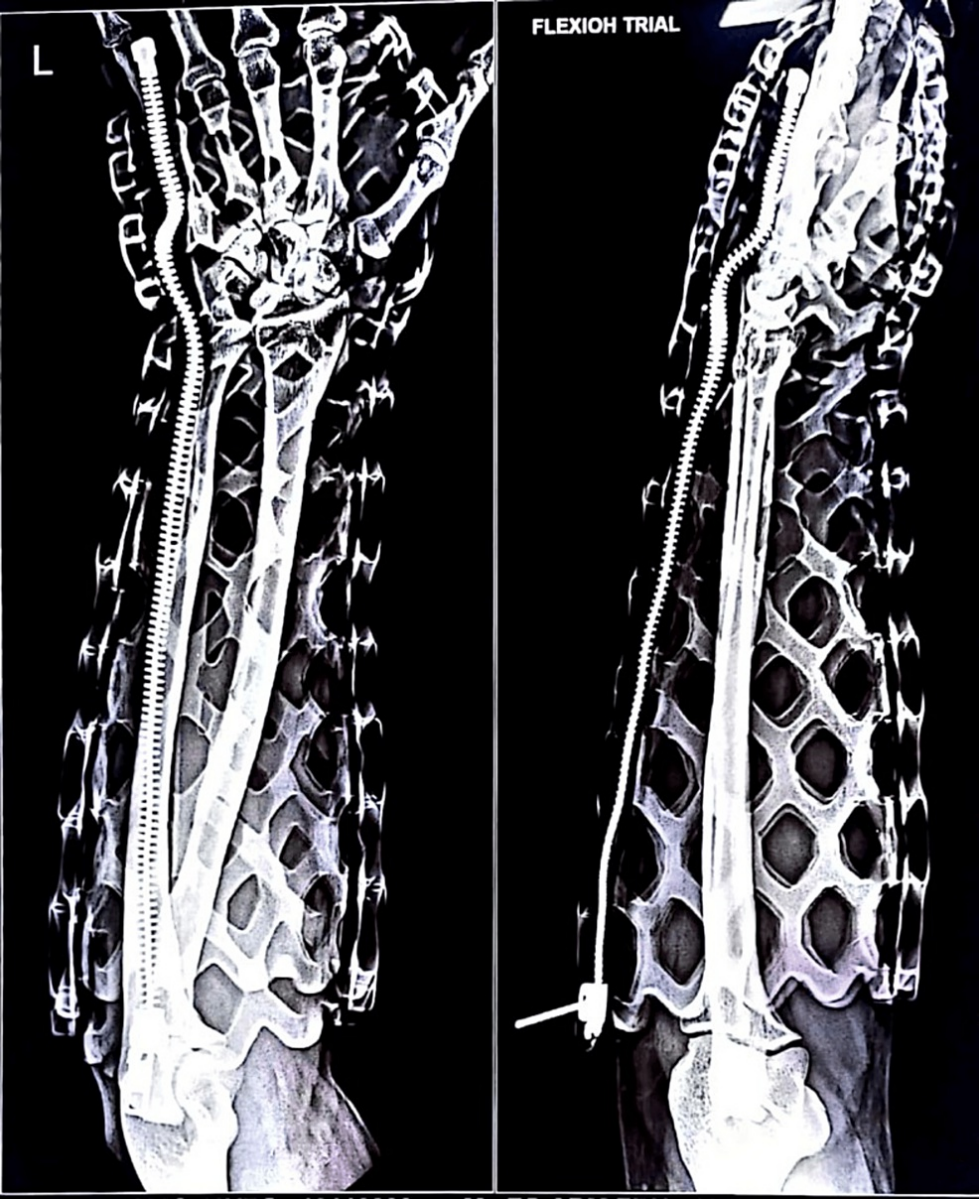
Radiograph of the short-arm FlexiOH immobilizer Permission was obtained from the original publishers to reproduce this figure [23].
